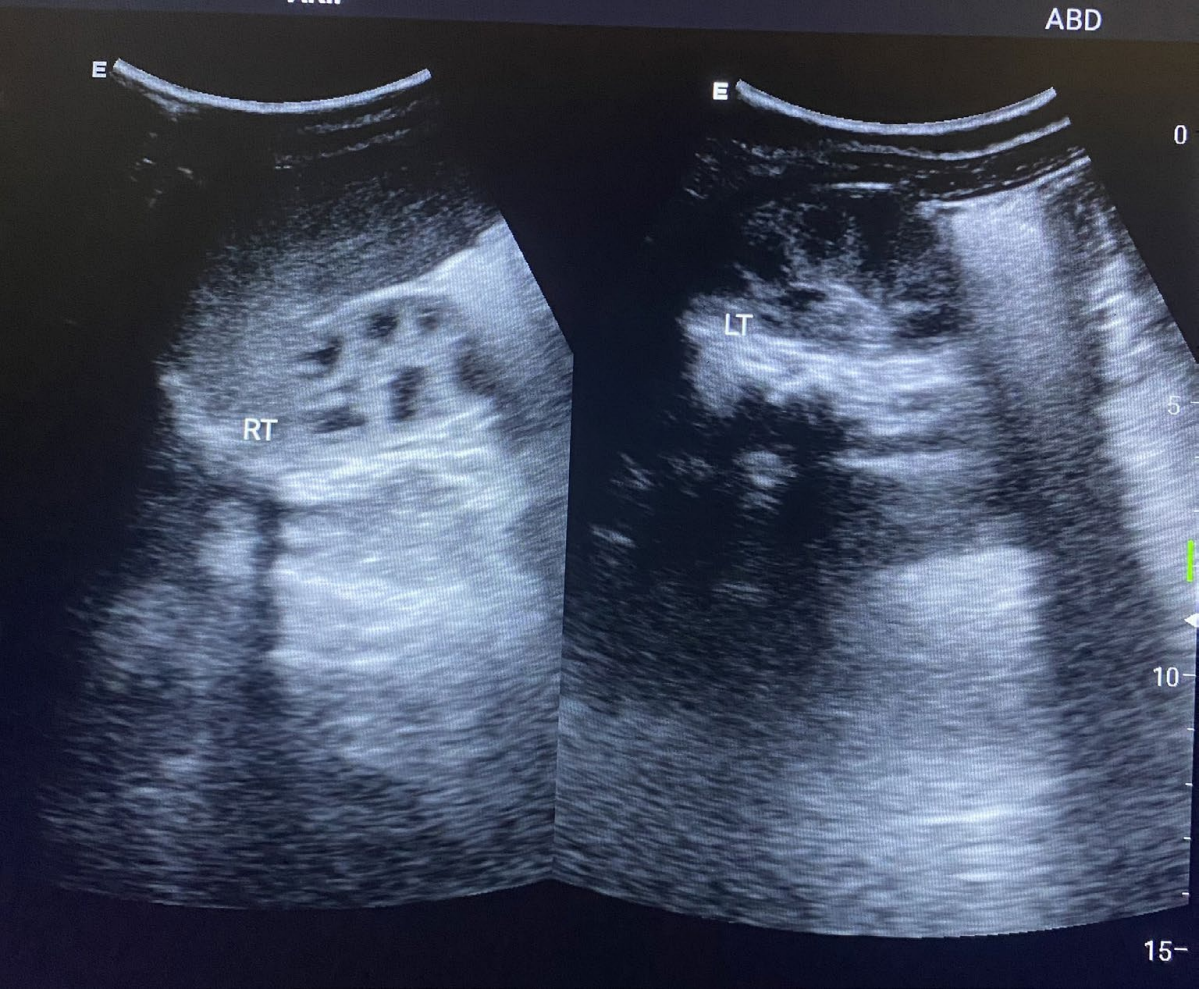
Ultrasonography after 6 weeks post‐delivery showing evidence of multiple cysts in the kidneys.
